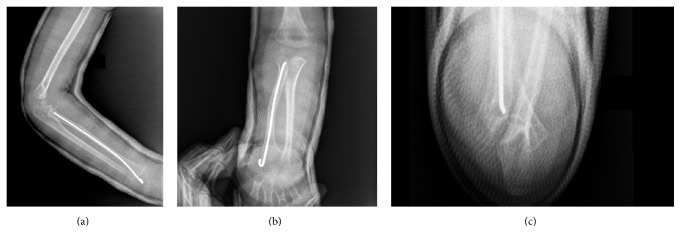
Postoperative radiographs show the radial head and anatomic ulnar reductions in lat (a), AP (b), and axial (c) views.
